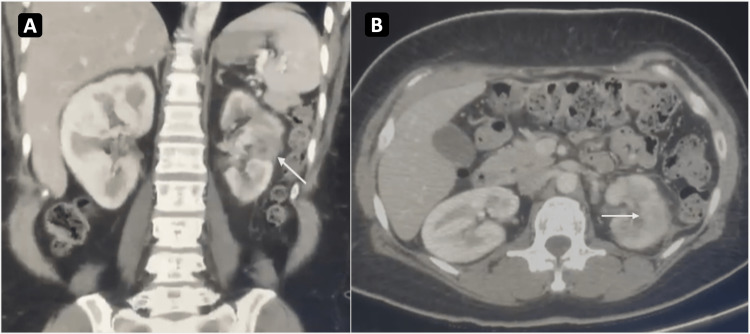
CT scan 2.5 months after discharge (A) Coronal section of enhanced CT of the abdomen with contrast revealed a left kidney interval decrease in size (white arrow) and resolution of previous hypodense lesions. (B) Cross-sectional view of enhanced CT of the abdomen revealed improved signs of emphysematous changes (white arrow). There was no air within the small residual collection.
